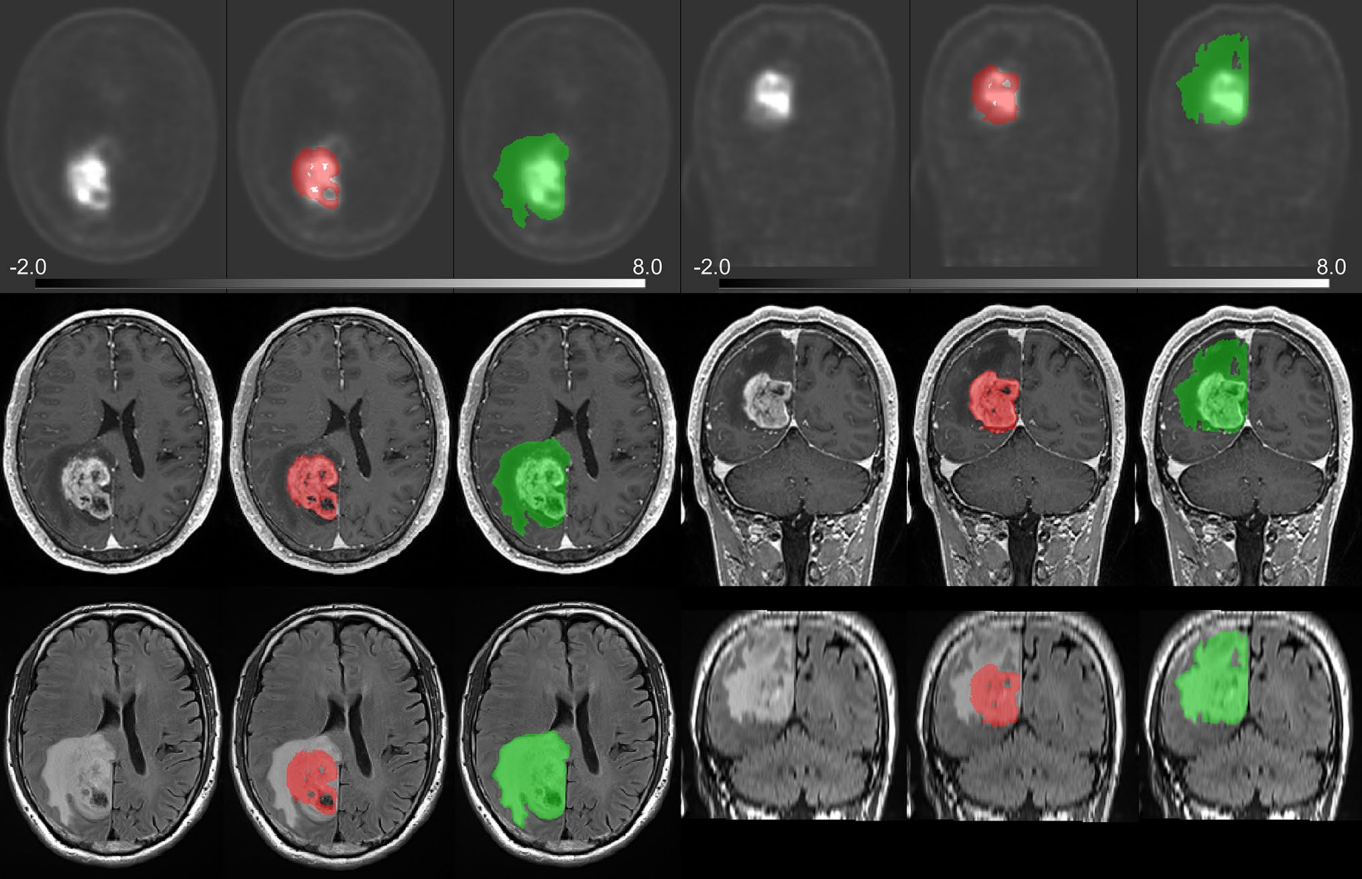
Representative VOIs for the case with Glioblastoma, *IDH*-wildtype are shown. FMISO (top), CE T1WI (middle row), FLAIR (bottom). Hyperintense areas on FLAIR imaging (HIA), representing areas of hyperintensity around and inside the tumor on FLAIR imaging (green), contrast-enhancing tumors (CET), representing tumor areas limited by the area of hyperintensity on CE T1WI (red)
